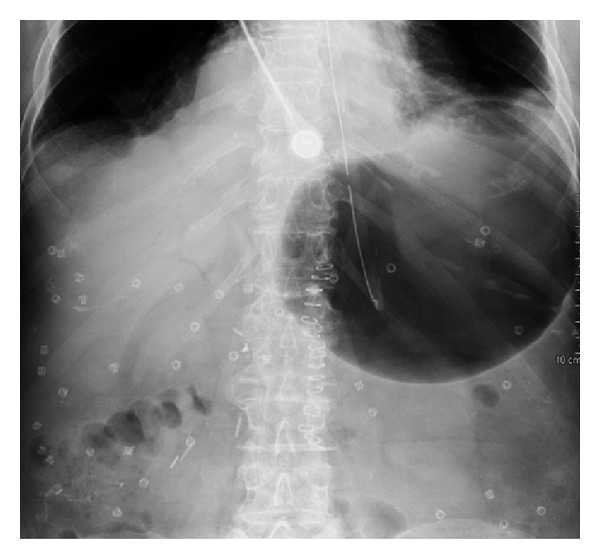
Plain film prior to contrast administration showing stomach distension.
